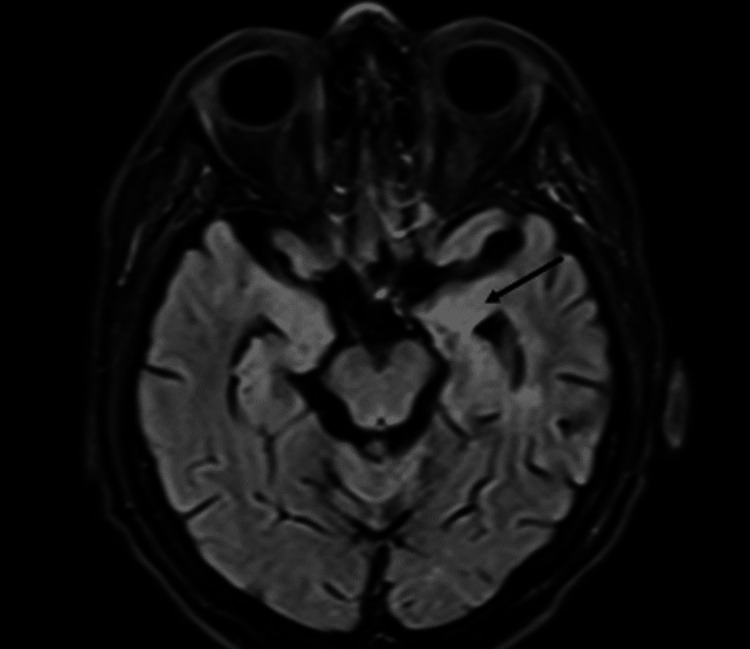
MRI from January 2024 showing the mesial region of the hippocampus with significant volume reduction and near-complete resolution of adjacent vasogenic edema compared to the previous study
